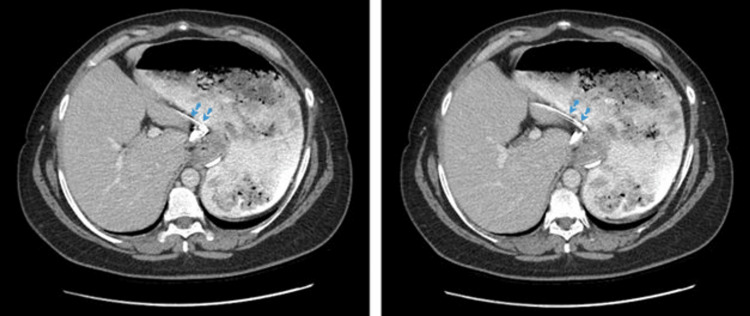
Computed tomography with contrast, axial plane. Proximal end of the catheter (blue dotted arrows).
